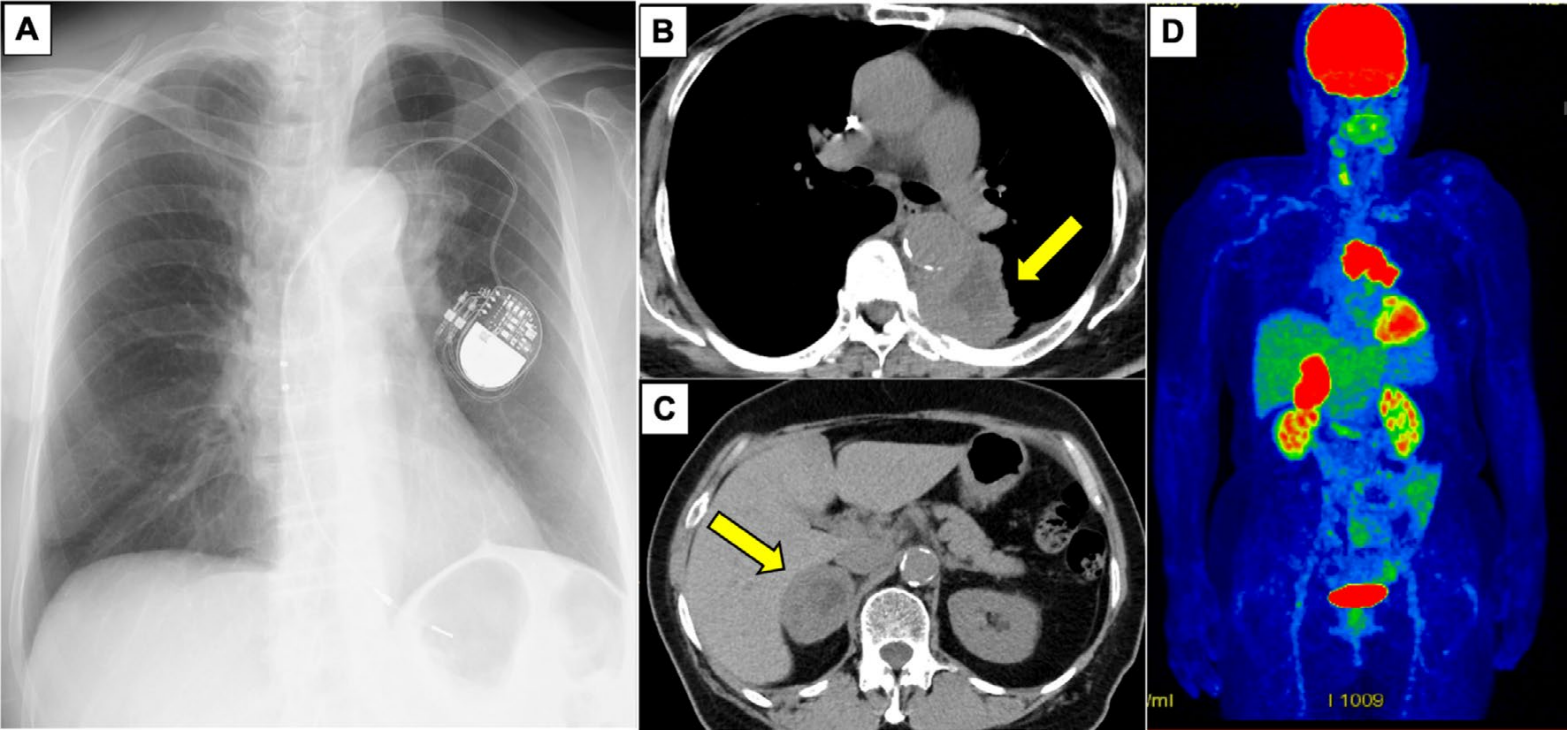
(A) Chest X‐ray image in the first visit to NHO Kochi Hospital in August 2023. A pacemaker was implanted and a mass shadow was present on the mediastinal side of the left lung field. (B and C) Computed tomography (CT) images taken during the first visit to NHO Kochi Hospital in August 2023. CT showed a mass shadow in the left lower lobe and right adrenal gland enlargement. (D) Positron emission tomography‐CT shows ^18^F‐fluorodeoxyglucose (FDG) uptake (the maximum standardized uptake value [SUVmax = 14.4]) in the mass of the left lower lobe and FDG uptake (SUVmax = 13.7) in the right adrenal gland.
